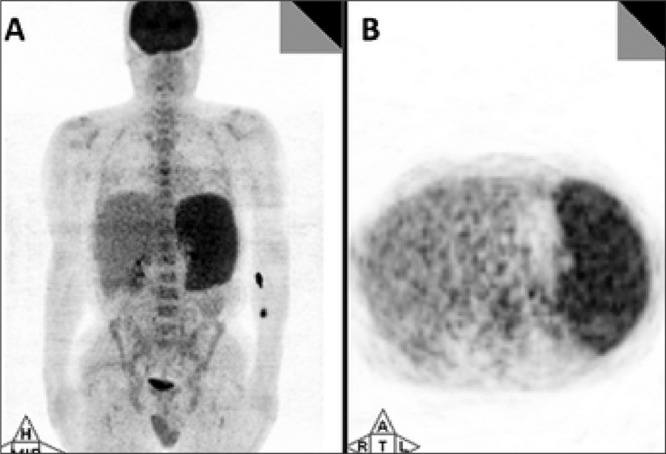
Anterior MIP (A) and transaxial (B) PET images showhepatosplenomegaly and a diffuse involvement of spleen. After PET/CT scanseveral new smears were prepared and diagnosed as having babesiosis
